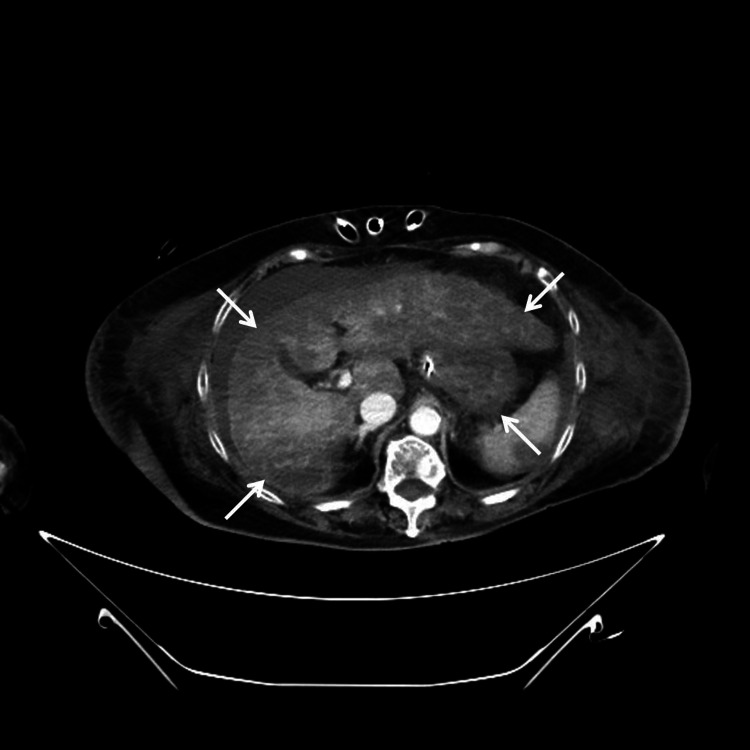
Contrast-enhanced CT, transverse section of the liver Contrast-enhanced computed tomography (CT) showed diffuse areas of non-enhancement in the liver (white arrows).
